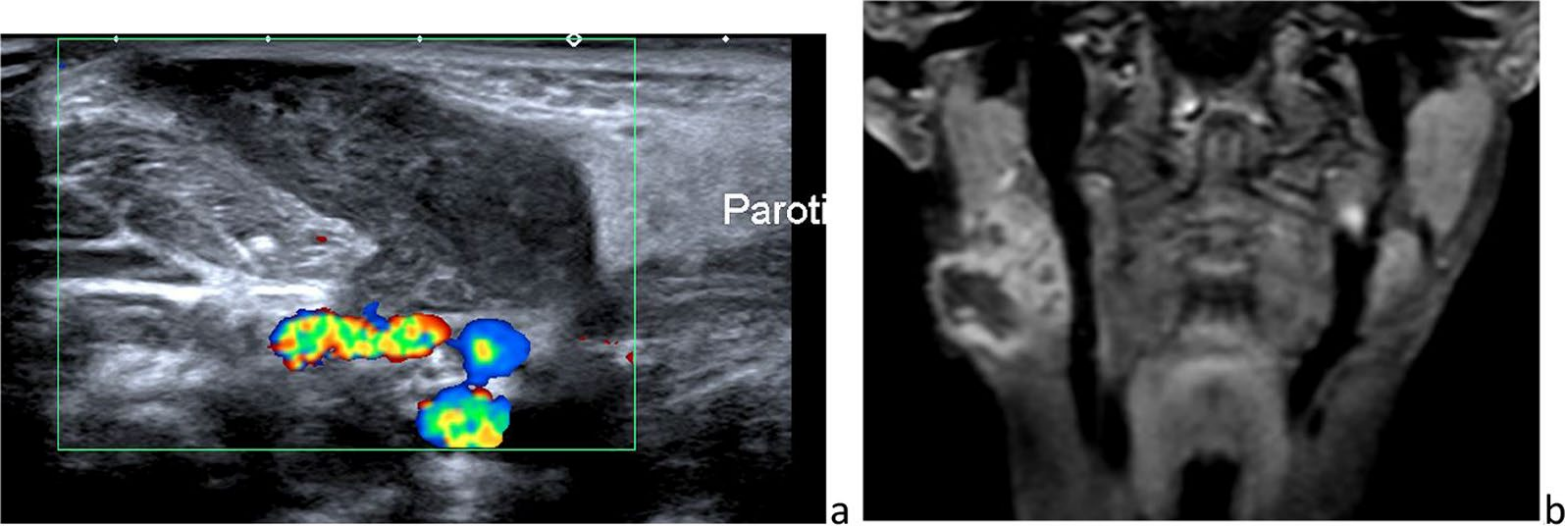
Mycobacterial infection. Necrotic lymphadenitis located immediately below the parotid gland and complicated by a skin fistula: sonography (a) and frontal T_1_-weighted image, after intravenous administration of gadolinium chelate (b)
